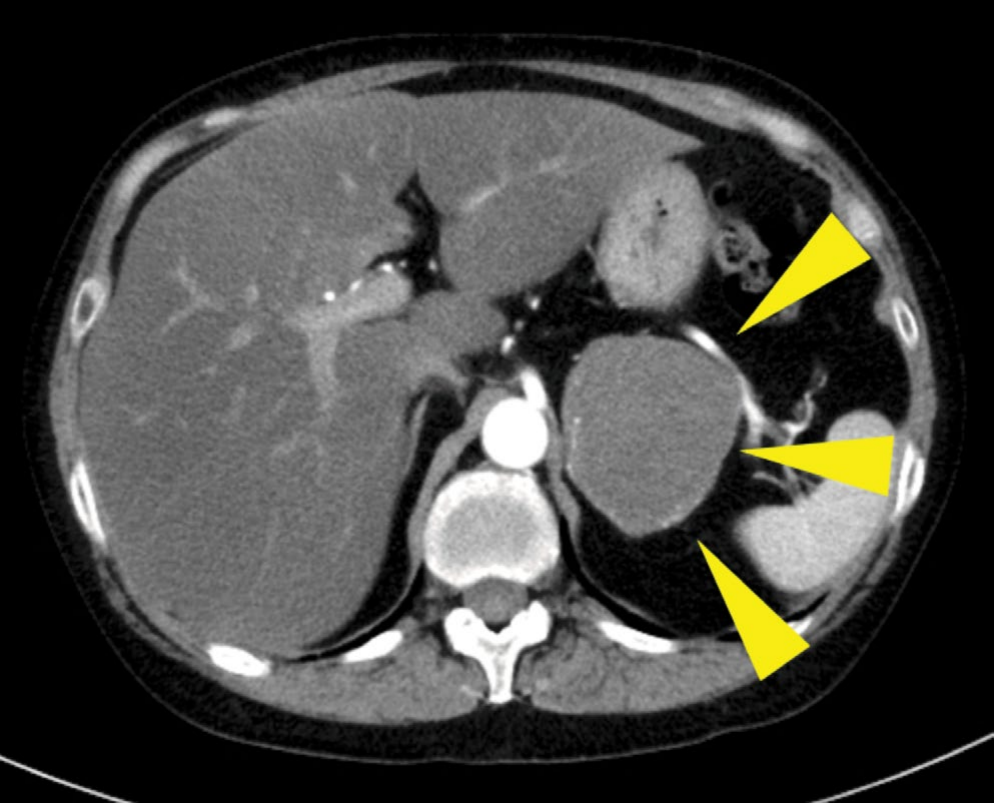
Initial contrast‐enhanced CT demonstrating a well‐demarcated 60‐mm left adrenal mass without surrounding organ invasion.
